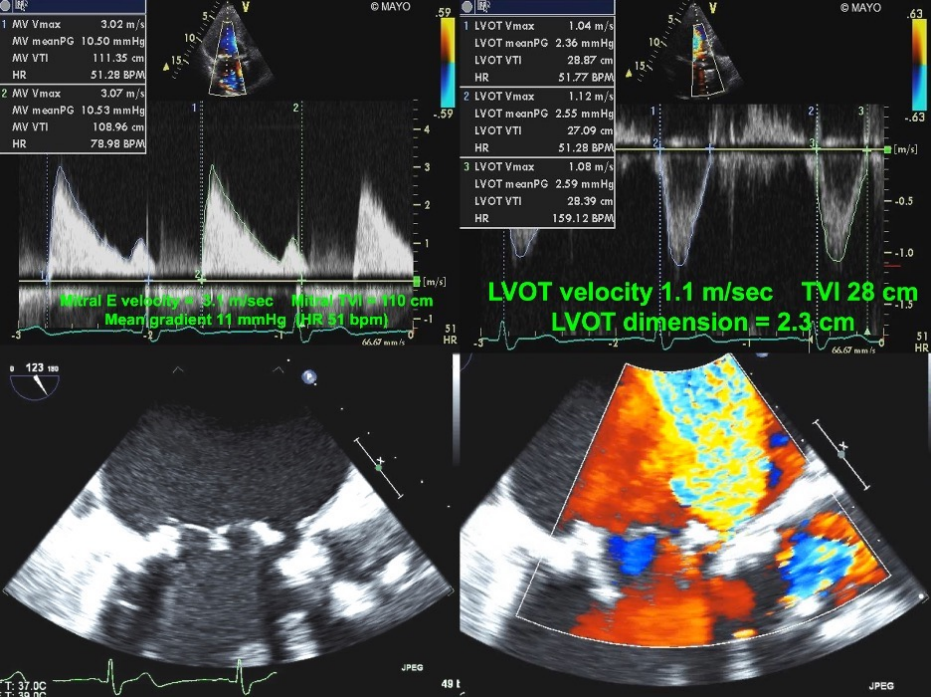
Echocardiographic imaging of a patient with a mitral bioprosthesis and prosthetic dehiscence. *Top Left and Right*: Continuous Wave Doppler of a male
